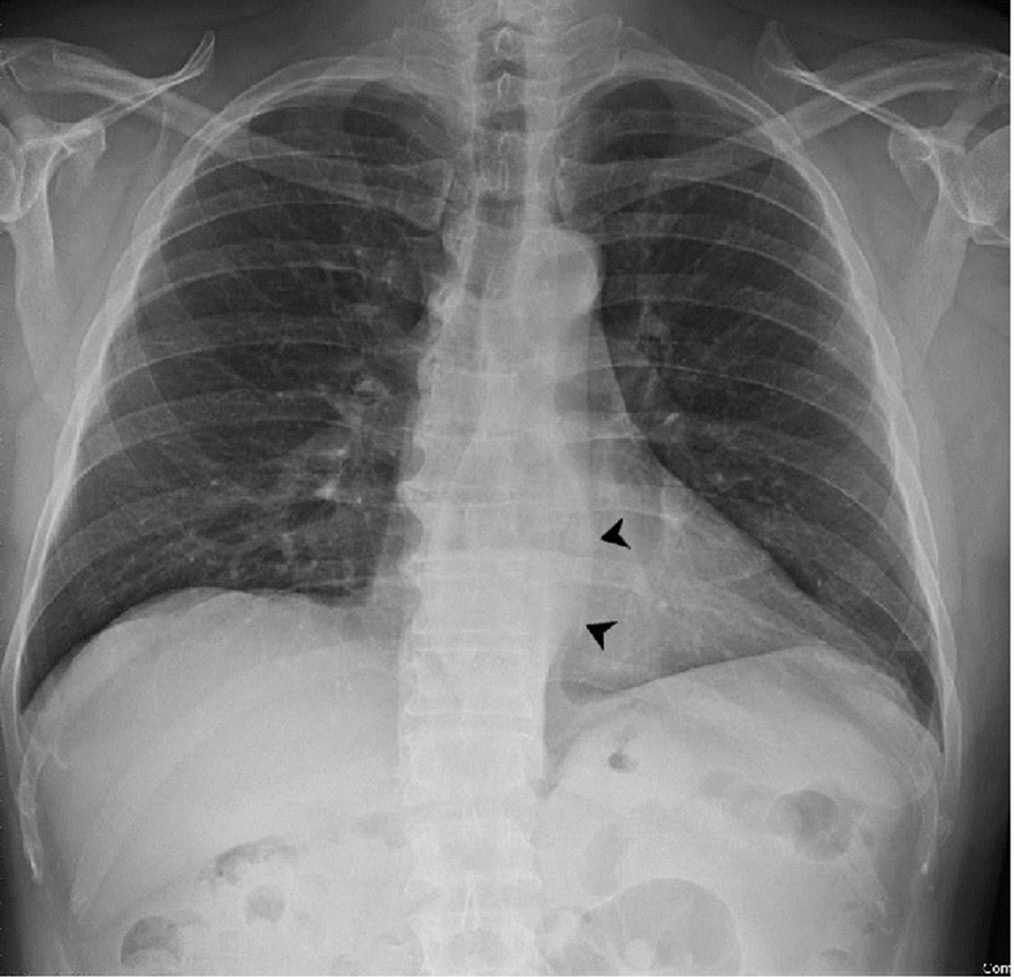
Pre-operative chest X-ray showing the wall of the diverticulum (arrowheads).
